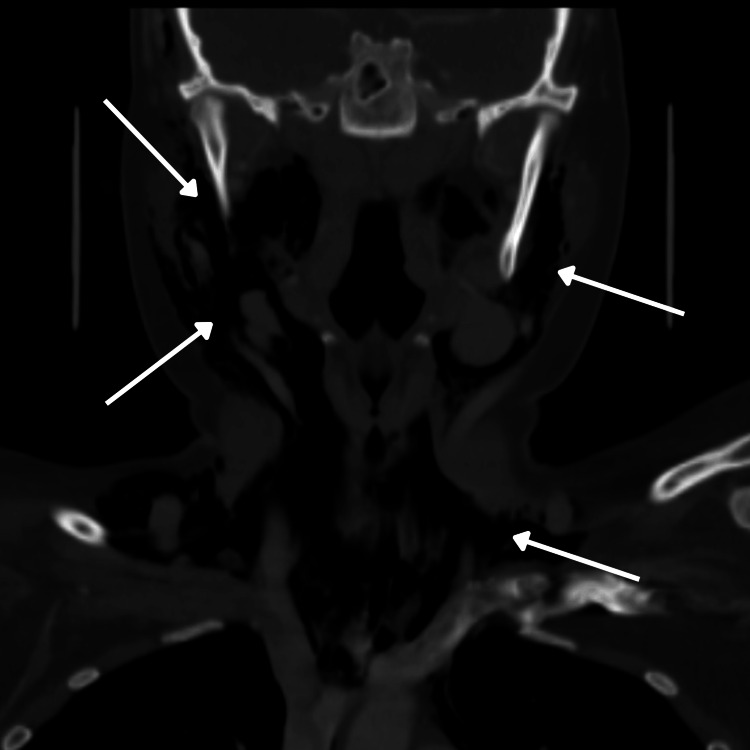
Coronal contrast-enhanced CT of the neck and thorax Gas (arrows) extends in continuity from the cervicofacial soft tissues through the thoracic inlet into the mediastinum. CT: computed tomography
